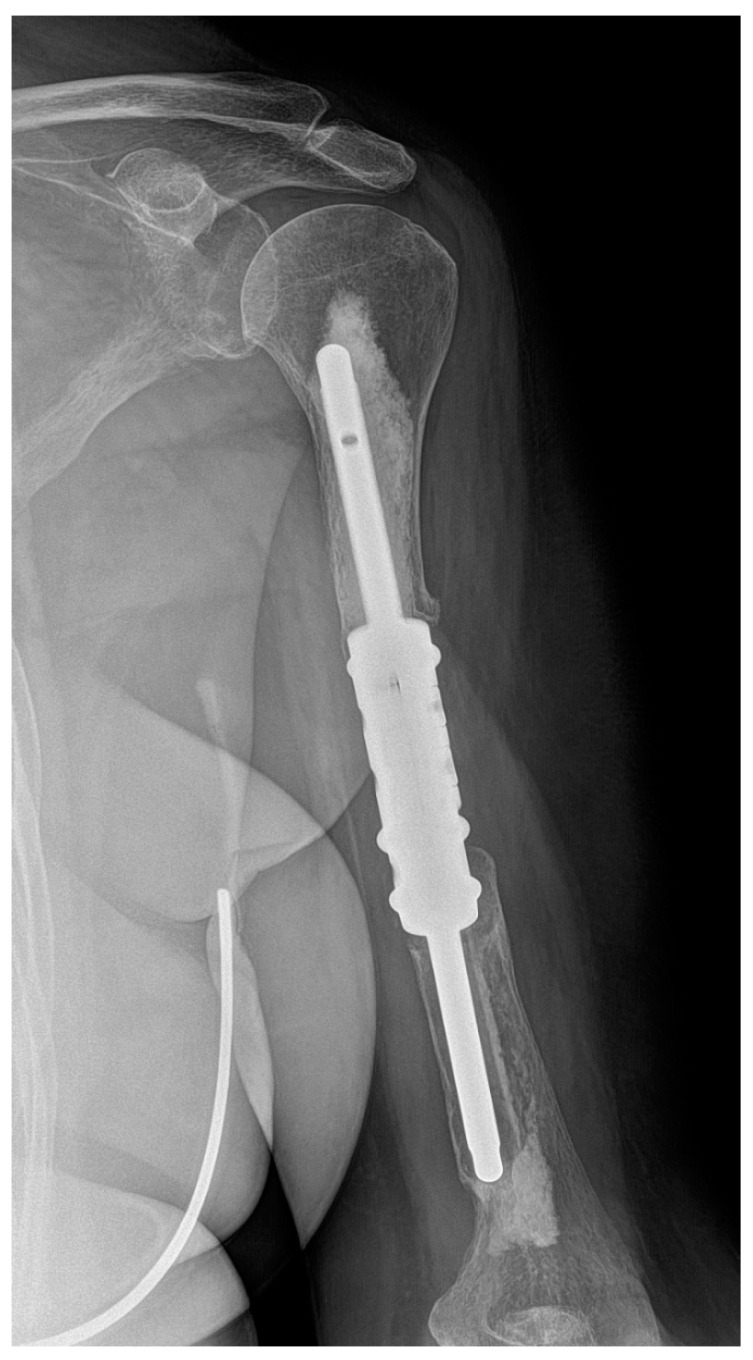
X-ray image of aseptic loosening of the distal stem of the humeral intercalary endoprosthesis. Resection of the metastasis revealed it was a uterine sarcoma solitary metastatic lesion of the left humerus. After 23 months, there was aseptic loosening. Due to the patient’s poor general condition (pulmonary and multiple skeletal metastases) and acceptable functional result (a Musculoskeletal Tumor Society score of 21), revision was not indicated. This image is from patient 7 in Table 1.
